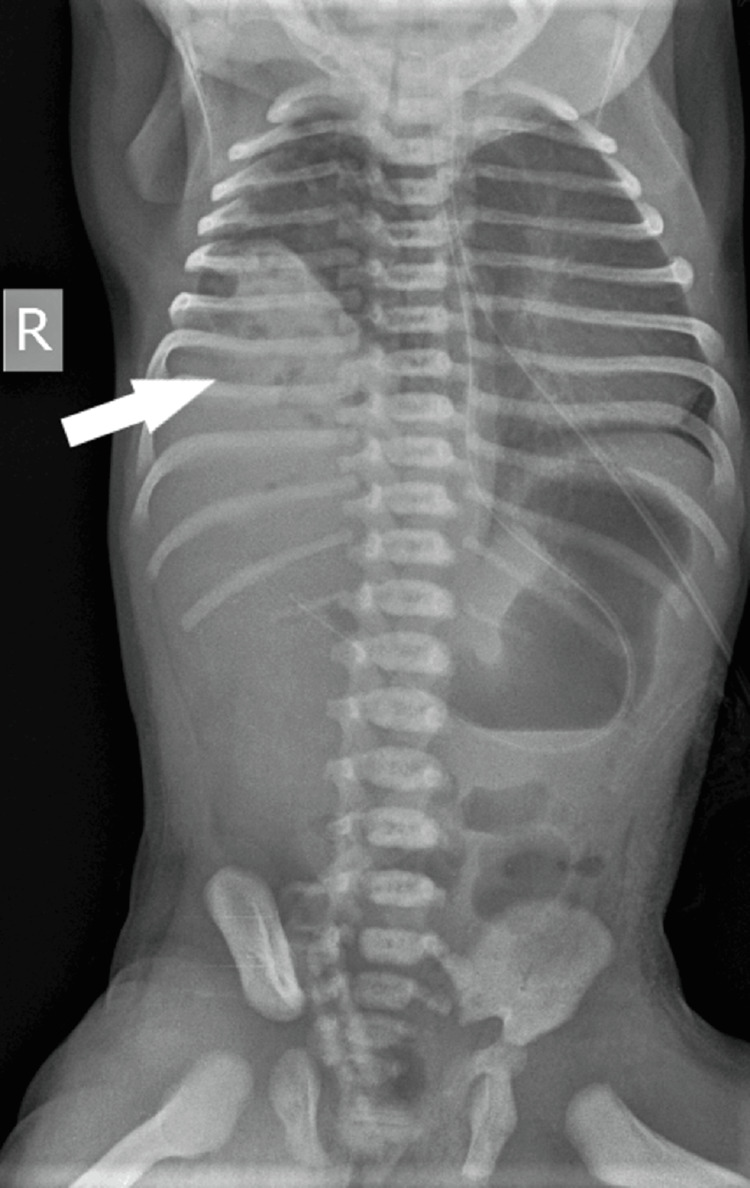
X-ray showing herniation of abdominal contents in the right hemithorax (arrow).
